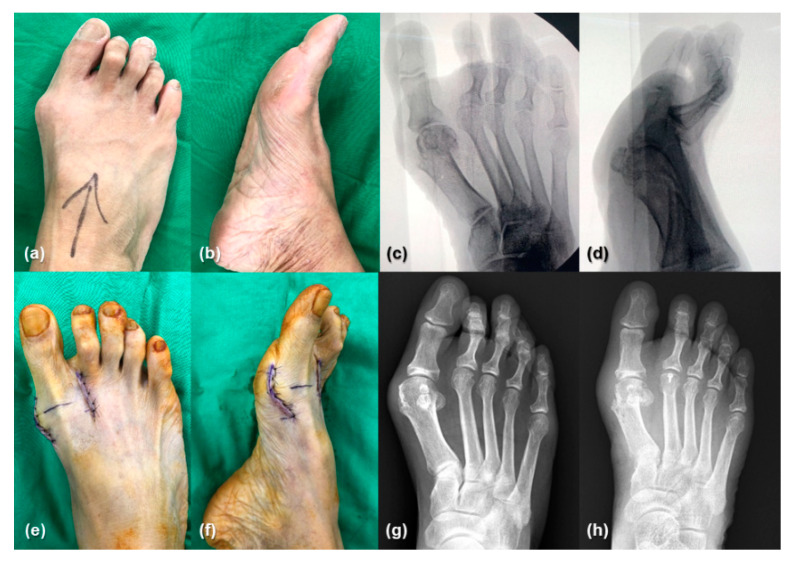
A 61-year-old woman presented with symptomatic hallux valgus. (a,b) Preoperative gross pictures depict hallux valgus deformity. (c) Anteroposterior and (d) lateral intraoperative C-arm fluoroscopy images depict restored alignment. (e,f) Postoperative gross pictures depict proper deformity correction. (g) A preoperative radiograph depicts an HVA of 38° and IMA of 15°. (h) A radiograph at 1 month after surgery depicts an HVA of 12° and IMA of 6°.
